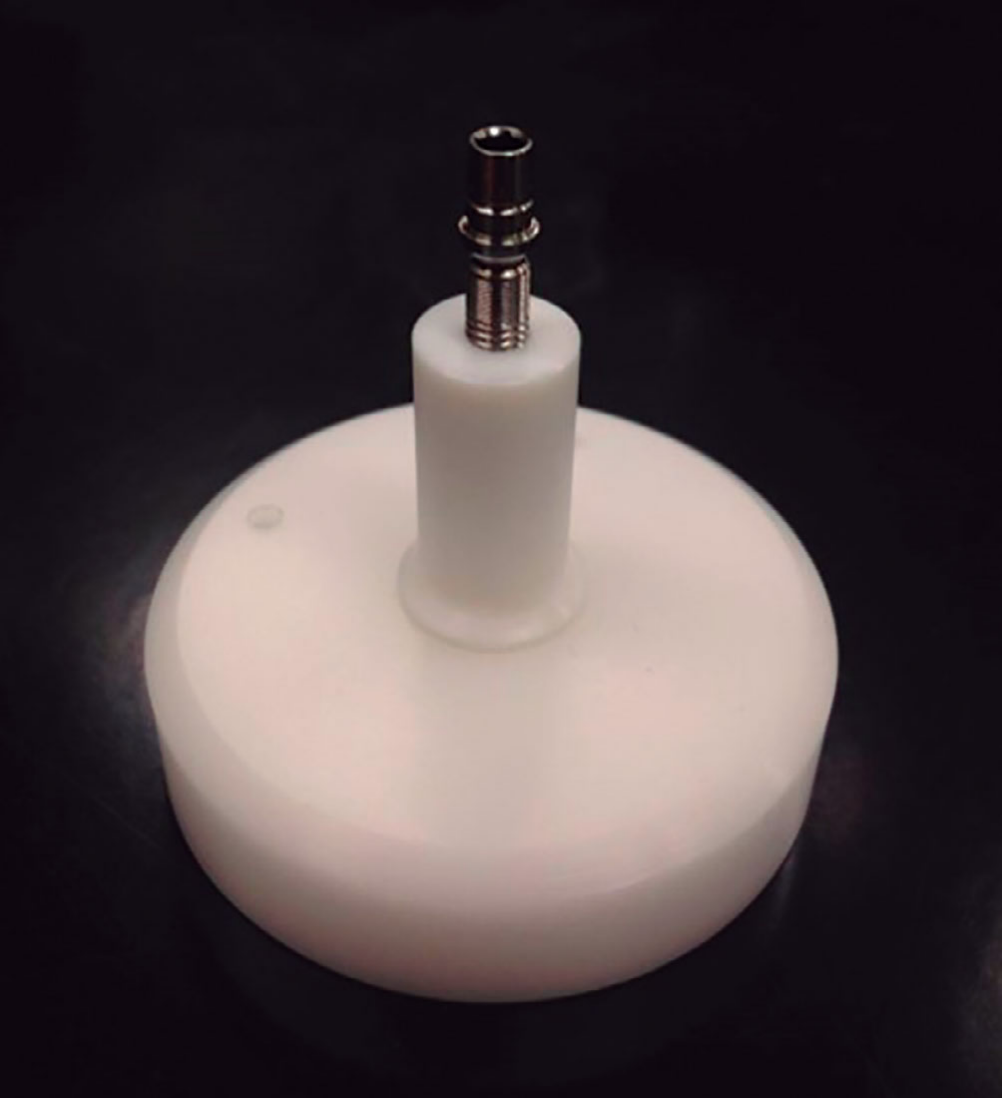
Teflon™ platform with implant mounted for micro‐CT
